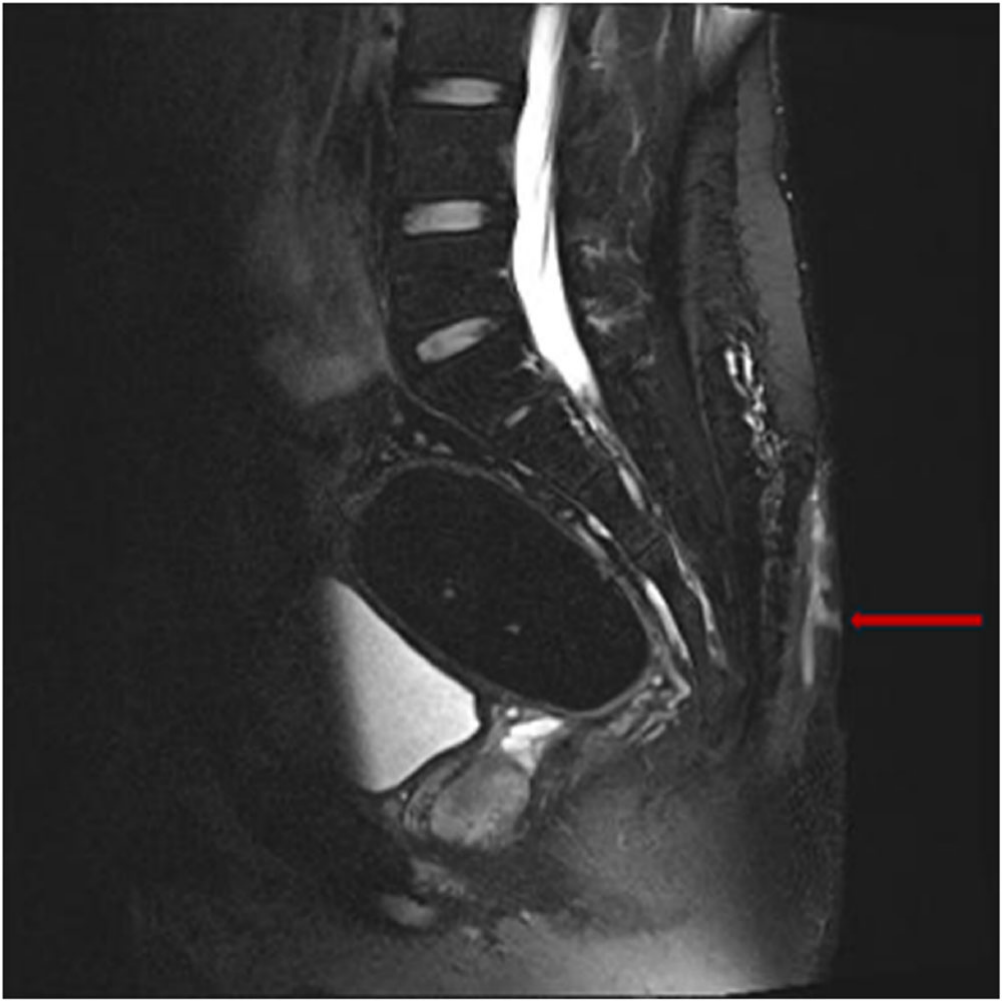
Sagittal MRI image demonstrating a complex PSD (red arrow). Courtesy of Prof. Jiong Wu.
